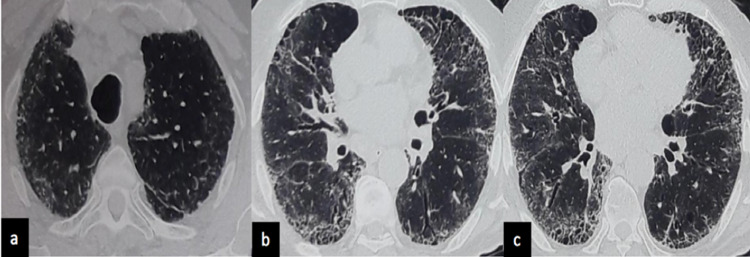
HRCT of the chest showing (a) inter-lobular, (b) intra-lobular, and (c) sub-pleural interstitial thickening involving the basal and peripheral regions, along with traction bronchiectasis and honeycombing. HRCT, high-resolution computed tomography
